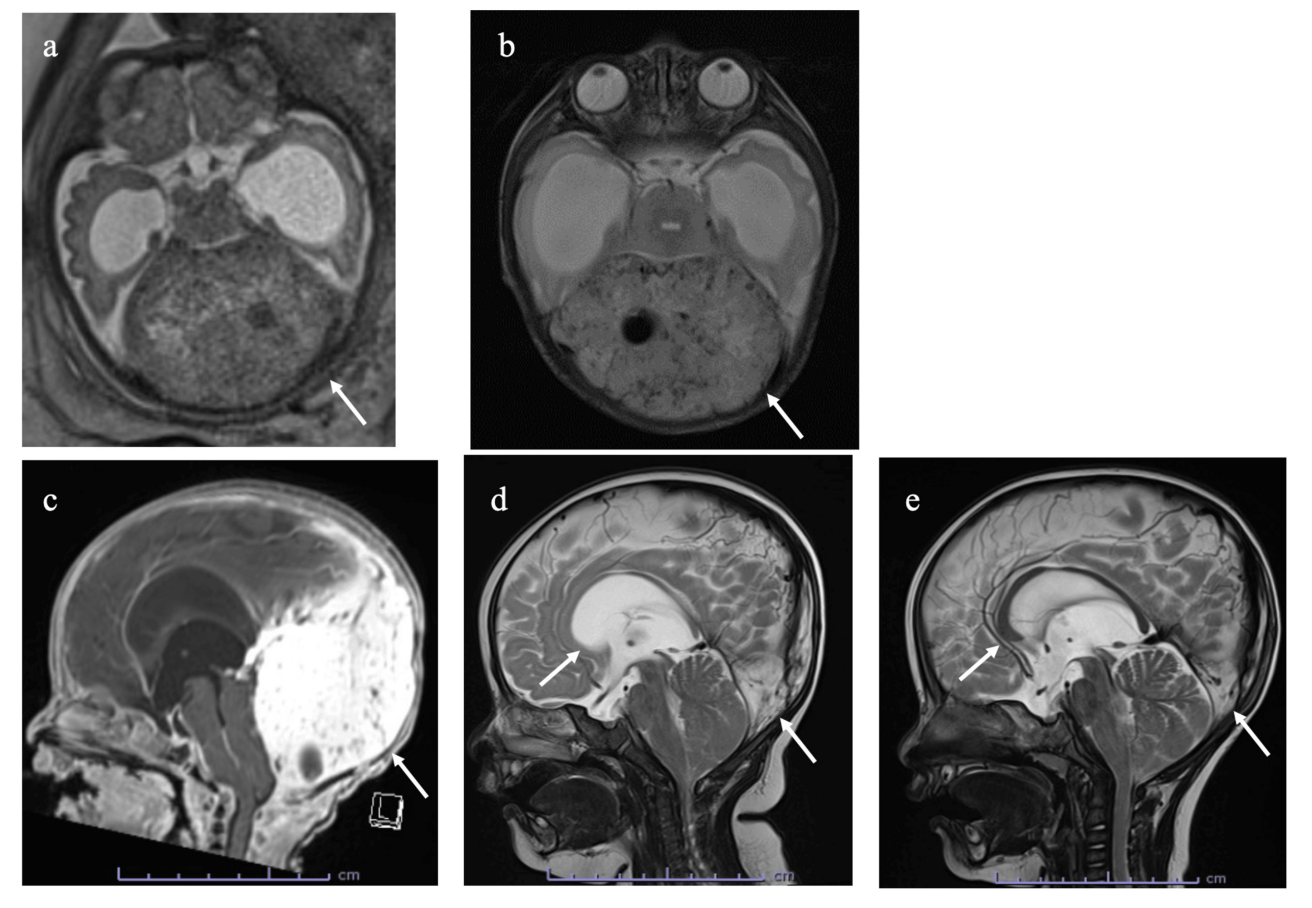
Magnetic resonance imaging (MRI) of the brain in the patient with congenital intracranial hemangioma (a) Fetal horizontal MRI at 32 weeks and 5 days of gestation, (b) horizontal MRI at 4 days old, (c) sagittal MRI at 4 days old, (d) sagittal MRI at 5 months after initiating oral propranolol treatment, and (e) sagittal MRI at 13 months after initiating oral propranolol treatment
